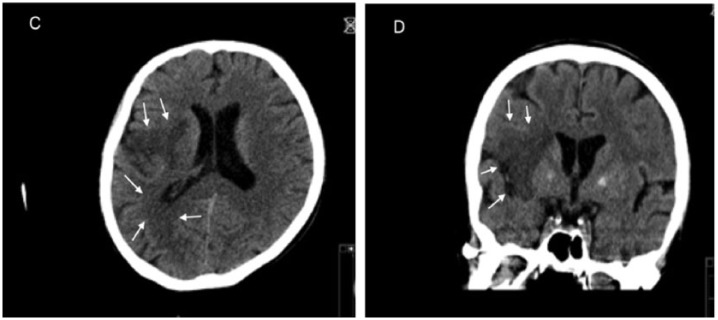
Computed tomography of the head—transverse plane (C) and frontal plane (D)—displaying hypodense areas (white arrows) consistent with subacute cerebral infarctions.
